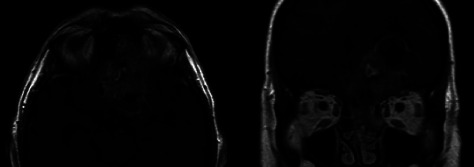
Axial and coronal postcontrast T1-weighted MR images of patient no. 2 with a T4bN0M0 tumor located in the left ethmoid sinus with gross intracranial extension. Note: extensive peritumoral (low signal intensity) edema resulting in a midline shift towards the right side.
